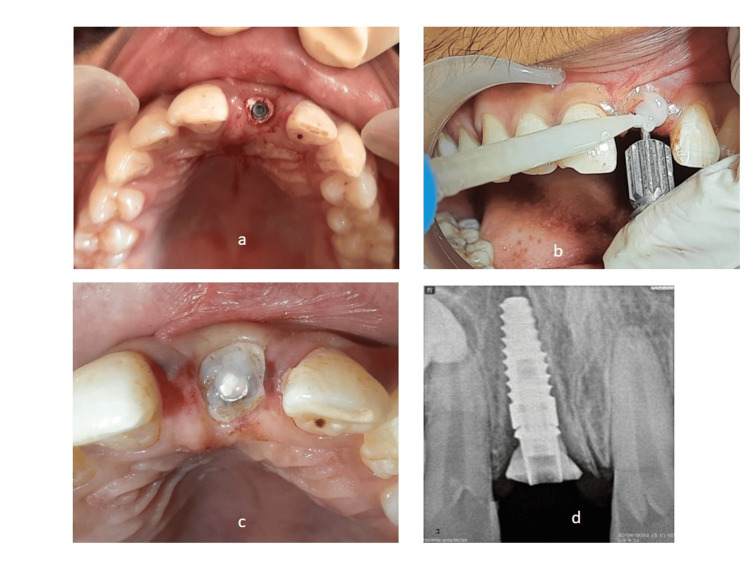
Fabrication of customized healing abutment (a) Space between the implant and soft tissue packed with Teflon; (b) Injecting bis-acrylic around abutment for customization; (c) Customized temporary abutment in place; (d) Radiographic evaluation to check complete seating of abutment
